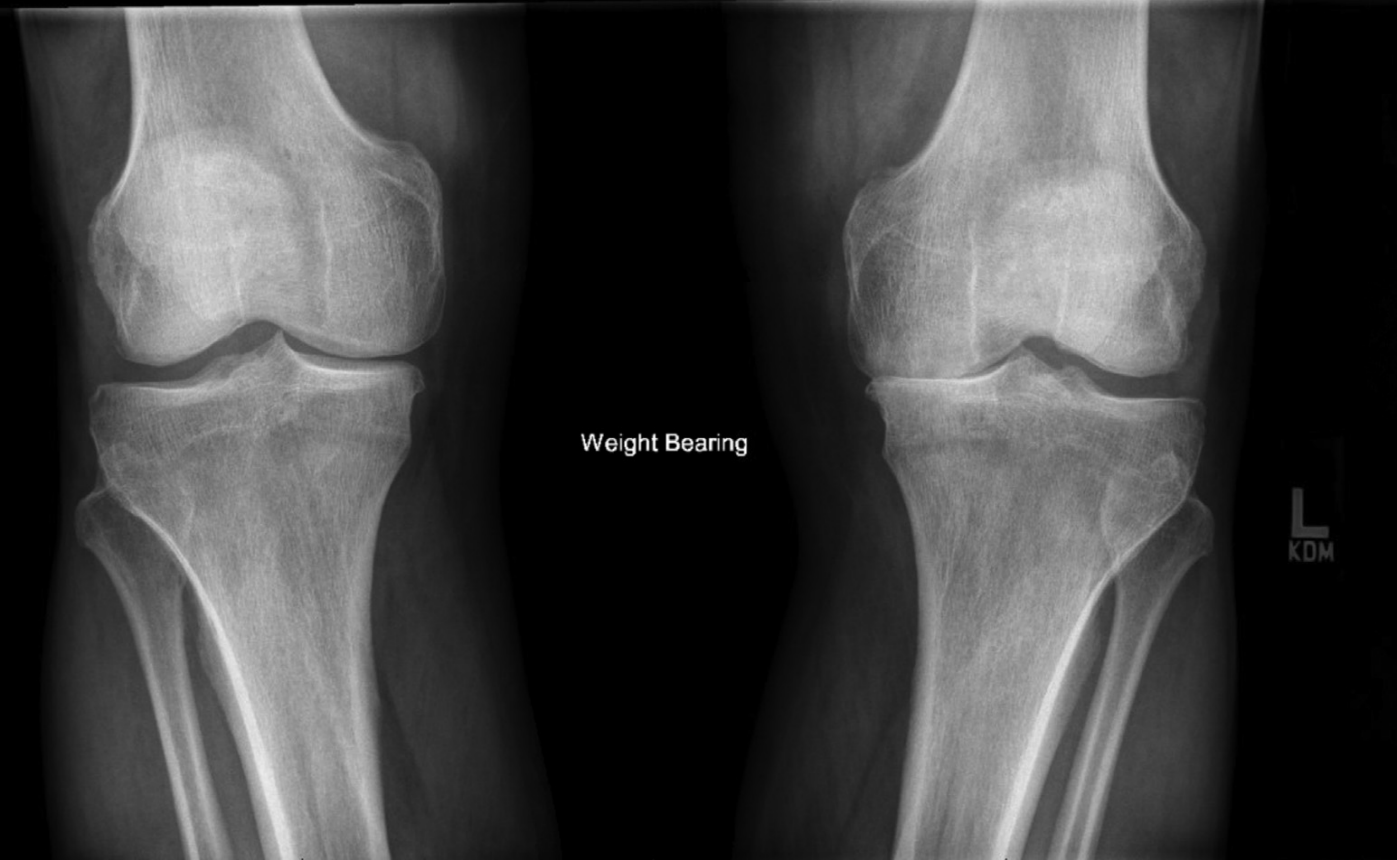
Bilateral standing anterior-posterior weight bearing knee radiographs.
